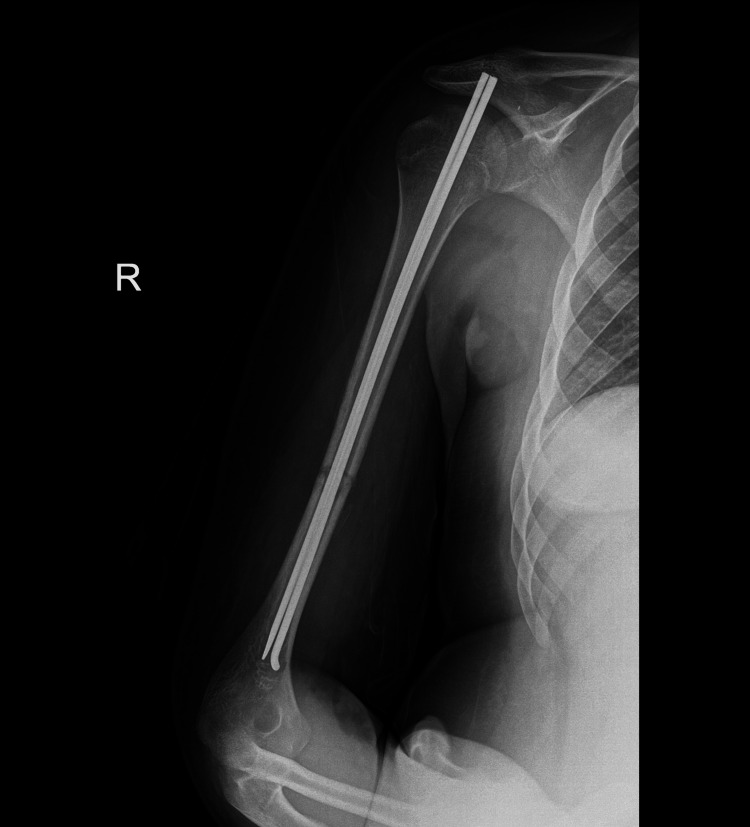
X-ray six weeks post-surgery, with healing signs
